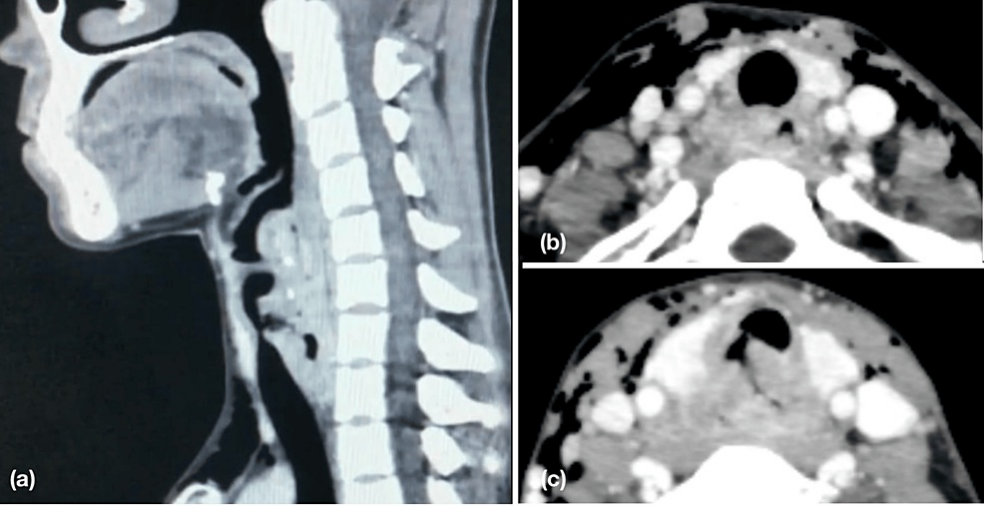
CT neck with contrast revealed an ill-defined heterogeneously enhancing soft tissue epicentered at the hypopharynx measuring 2 * 3 cm with soft tissue thickening extending from C5 to T1 vertebral level in sagittal view (a). The presence of subcutaneous emphysema and prevertebral space involvement in axial view (b). A suspicious communication of the lesion with the posterior wall of trachea and bulging soft tissue at the posterior wall of trachea in axial view (c).
